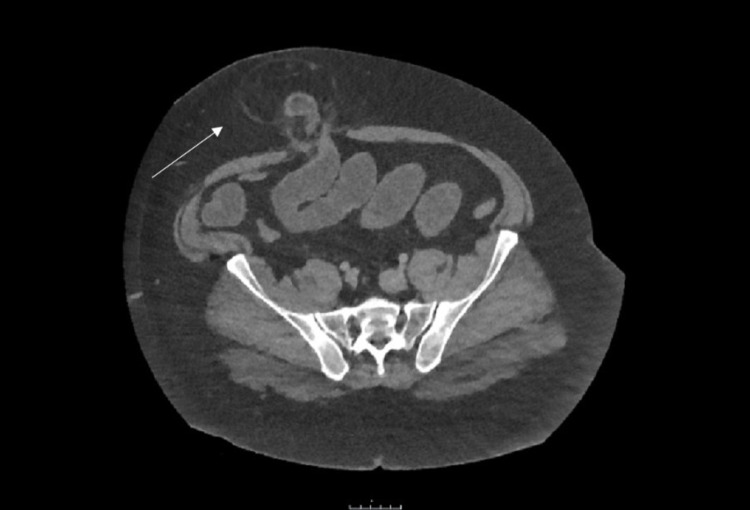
CT abdomen showing an axial view of paraumbilical hernia containing bowel, causing proximal small bowel dilatation.
